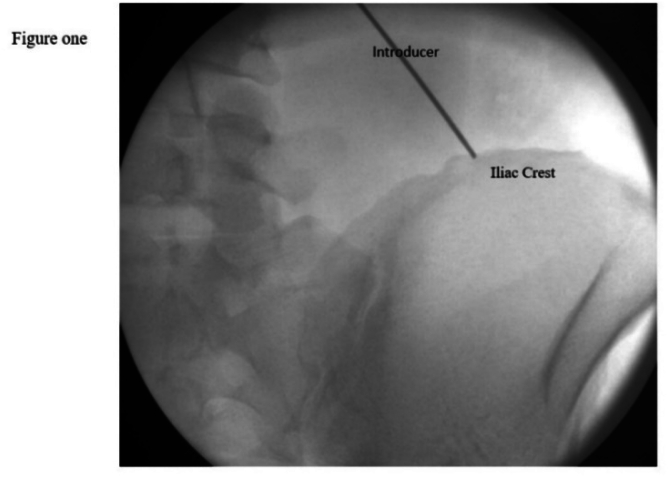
Xray AP View *Shows the introducer direction from medial cranial to caudal lateral with the tip above the iliac crest.*
